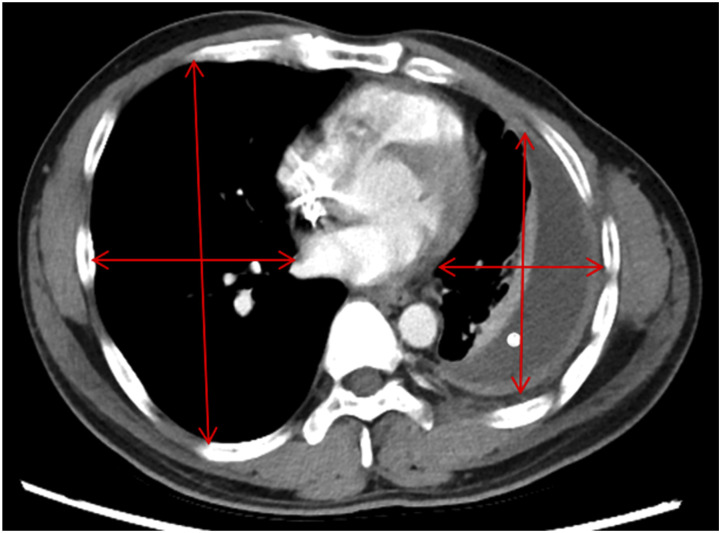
Quantitative assessment of thoracic morphological changes in patients with chronic TB empyema after pleural decortication using non-contrast chest computed tomography (CT). Preoperative CT shows right hemithoracic collapse, pleural thickening and calcification, right-sided loculated pleural effusion, and marked compression with poor expansion of the affected lung. Affected-side transverse diameter was measured from the inner edge of the most medial rib on the affected chest wall to the junction of the mediastinal pleura and the lung on the affected side. Unaffected-side transverse diameter was measured from the corresponding junction on the unaffected side to the inner edge of the most medial rib on the unaffected chest wall. Affected-side anteroposterior diameter was measured from the inner edge of the most anterior rib to the inner edge of the most posterior rib on the affected chest wall. Unaffected-side anteroposterior diameter was measured from the inner edge of the most anterior rib to the inner edge of the most posterior rib on the unaffected chest wall. The horizontal line indicates the transverse diameter, and the vertical line indicates the anteroposterior diameter.
